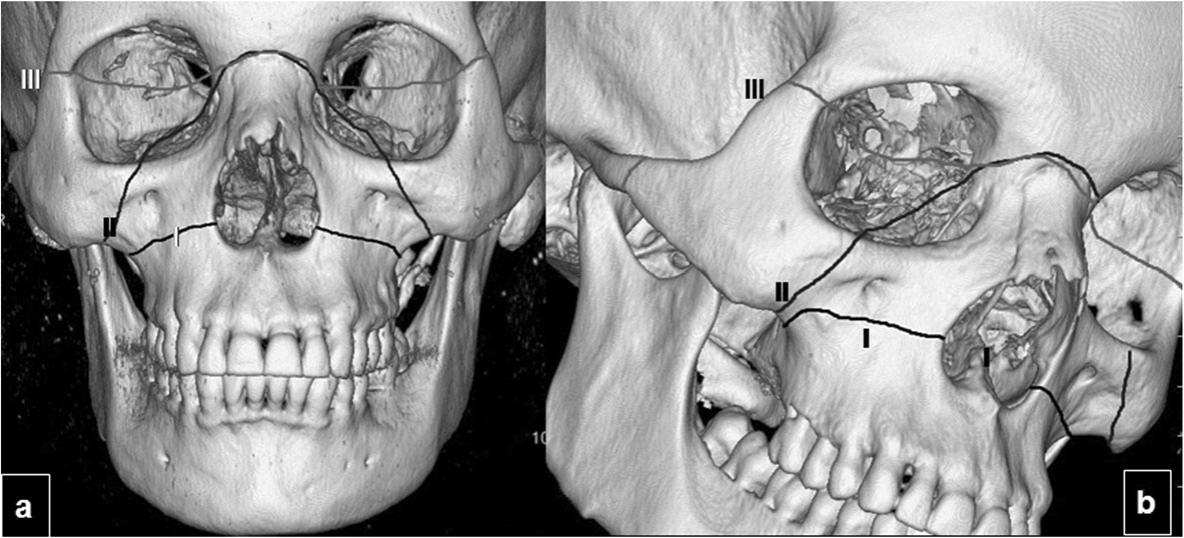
Le Fort fracture patterns. Three dimensional CT images of an adult skull in frontal (a) and oblique (b) orientations show the osseous facial structures classically affected in type I, II, and III Le Fort fractures
